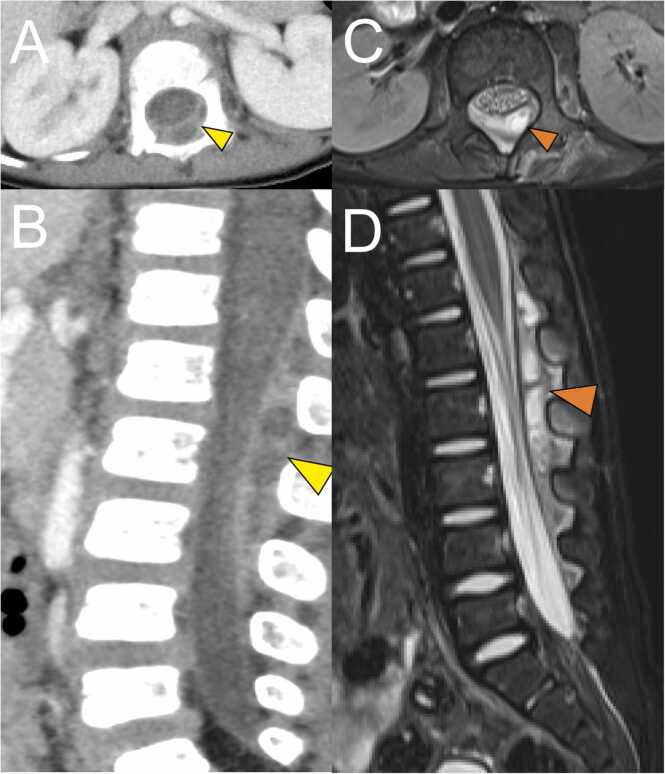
Contrast enhanced computed tomography (CT) imaging and magnetic resonance imaging (MRI) performed using a 3.0 T system. (A) Axial CT image. (B) Sagittal CT image. (C) Axial fat-suppressed T2-weighted MRI image. (D) Sagittal fat-suppressed T2-weighted MRI image. A multifocal cystic structure is visible dorsally on the dura at the Th12-L3 level in the spinal canal (yellow arrowhead in A, B; orange arrowhead in C, D). Contrast effects are present on the left lateral intervertebral foramen at the L1–2 level and on the erector spinae near the L1/2 intervertebral joint (A, B). A markedly high fat-suppressed T2-weighted image signal in the left intervertebral joint at the level of L1/2 is suggestive of fluid retention (C, D). The perivertebral foramen and erector spinae show high fat-suppressed T2-weighted image signals mainly at the L2 level, indicating inflammatory spillover (C, D). No abscess formation in the psoas major muscle or bone marrow edema in the vertebral body is visible.
